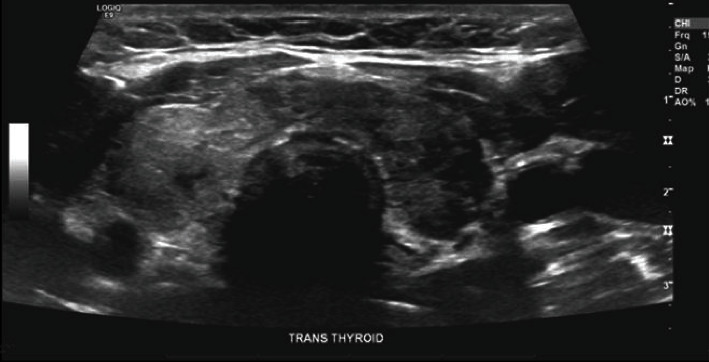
Ultrasound of the thyroid gland showing a heterogeneously enlarged thyroid gland with two small 4 mm solid hypoechoic solid nodules in the isthmus.
